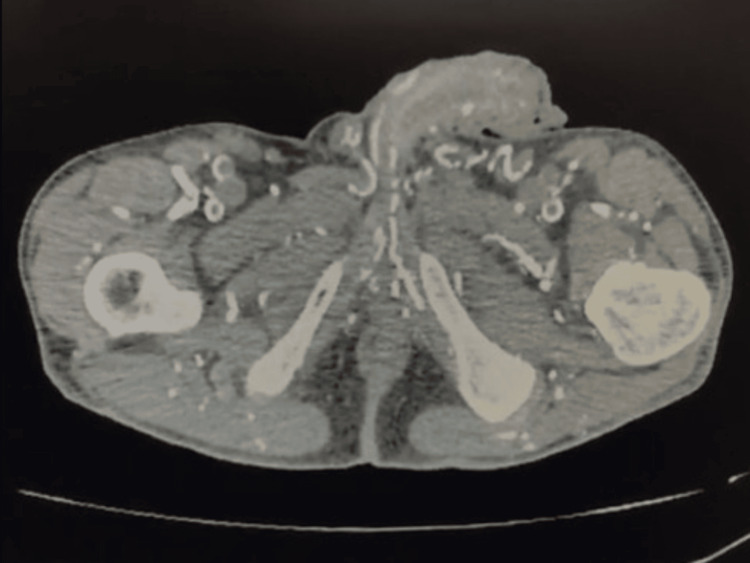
Simple CT scan (axial section) showing extensive calcification of the pelvic and genital vessels.
